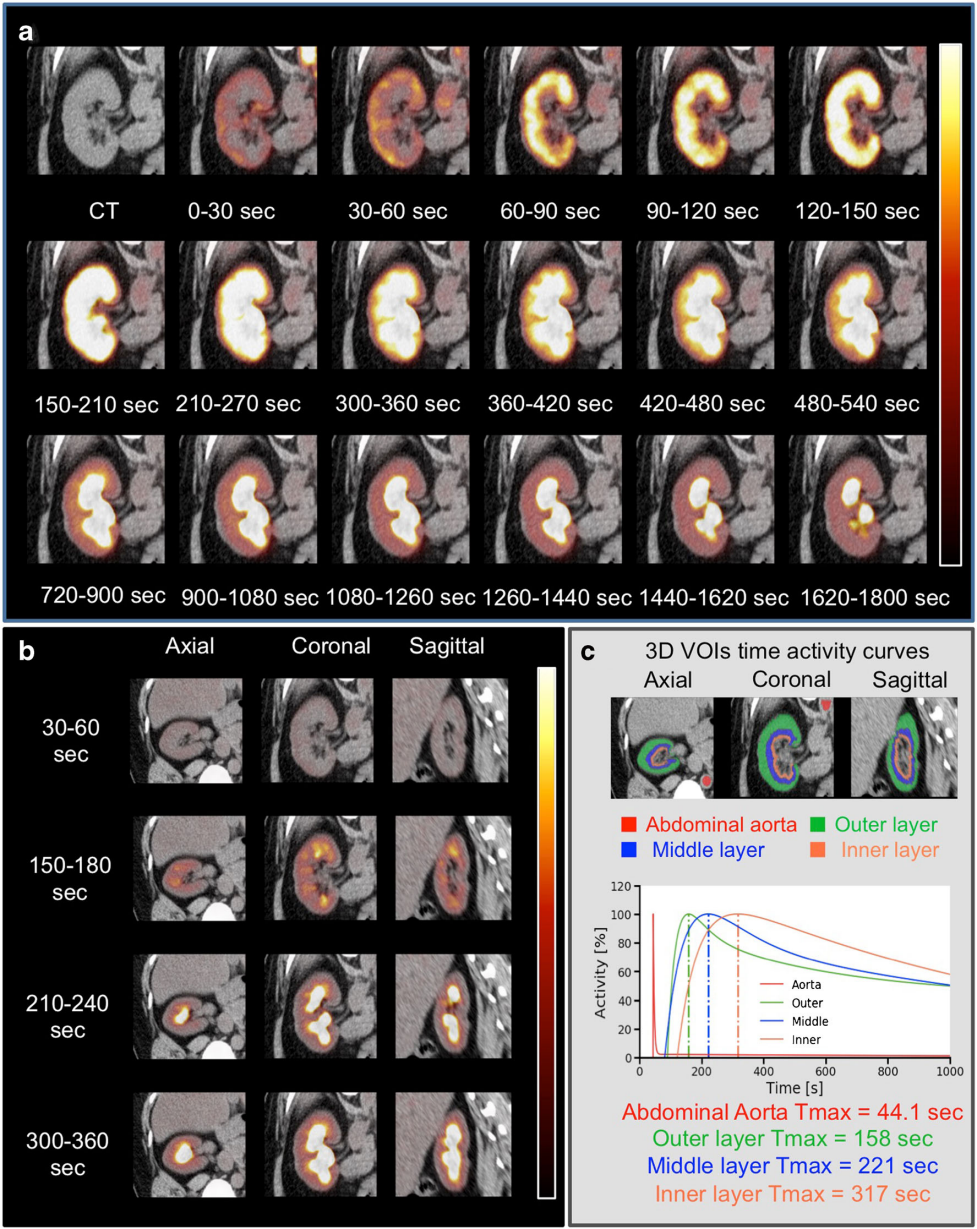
[^18^F]FDS PET/CT imaging of the right kidney in a 48-year old female volunteer. Dynamic coronal images (a) and axial, coronal and sagittal images (b) show rapid radiotracer accumulation in the renal cortex, followed by radiotracer excretion. c Average time–activity curves obtained by dynamic PET imaging. Three-dimensional (3D) volumes of interest (VOIs) were placed on the outer layer (corresponding to the cortex, *green*) as well as on the middle layer (*blue*) and inner layer (*orange*) corresponding to the medulla. Modified from Werner et al. [40]
